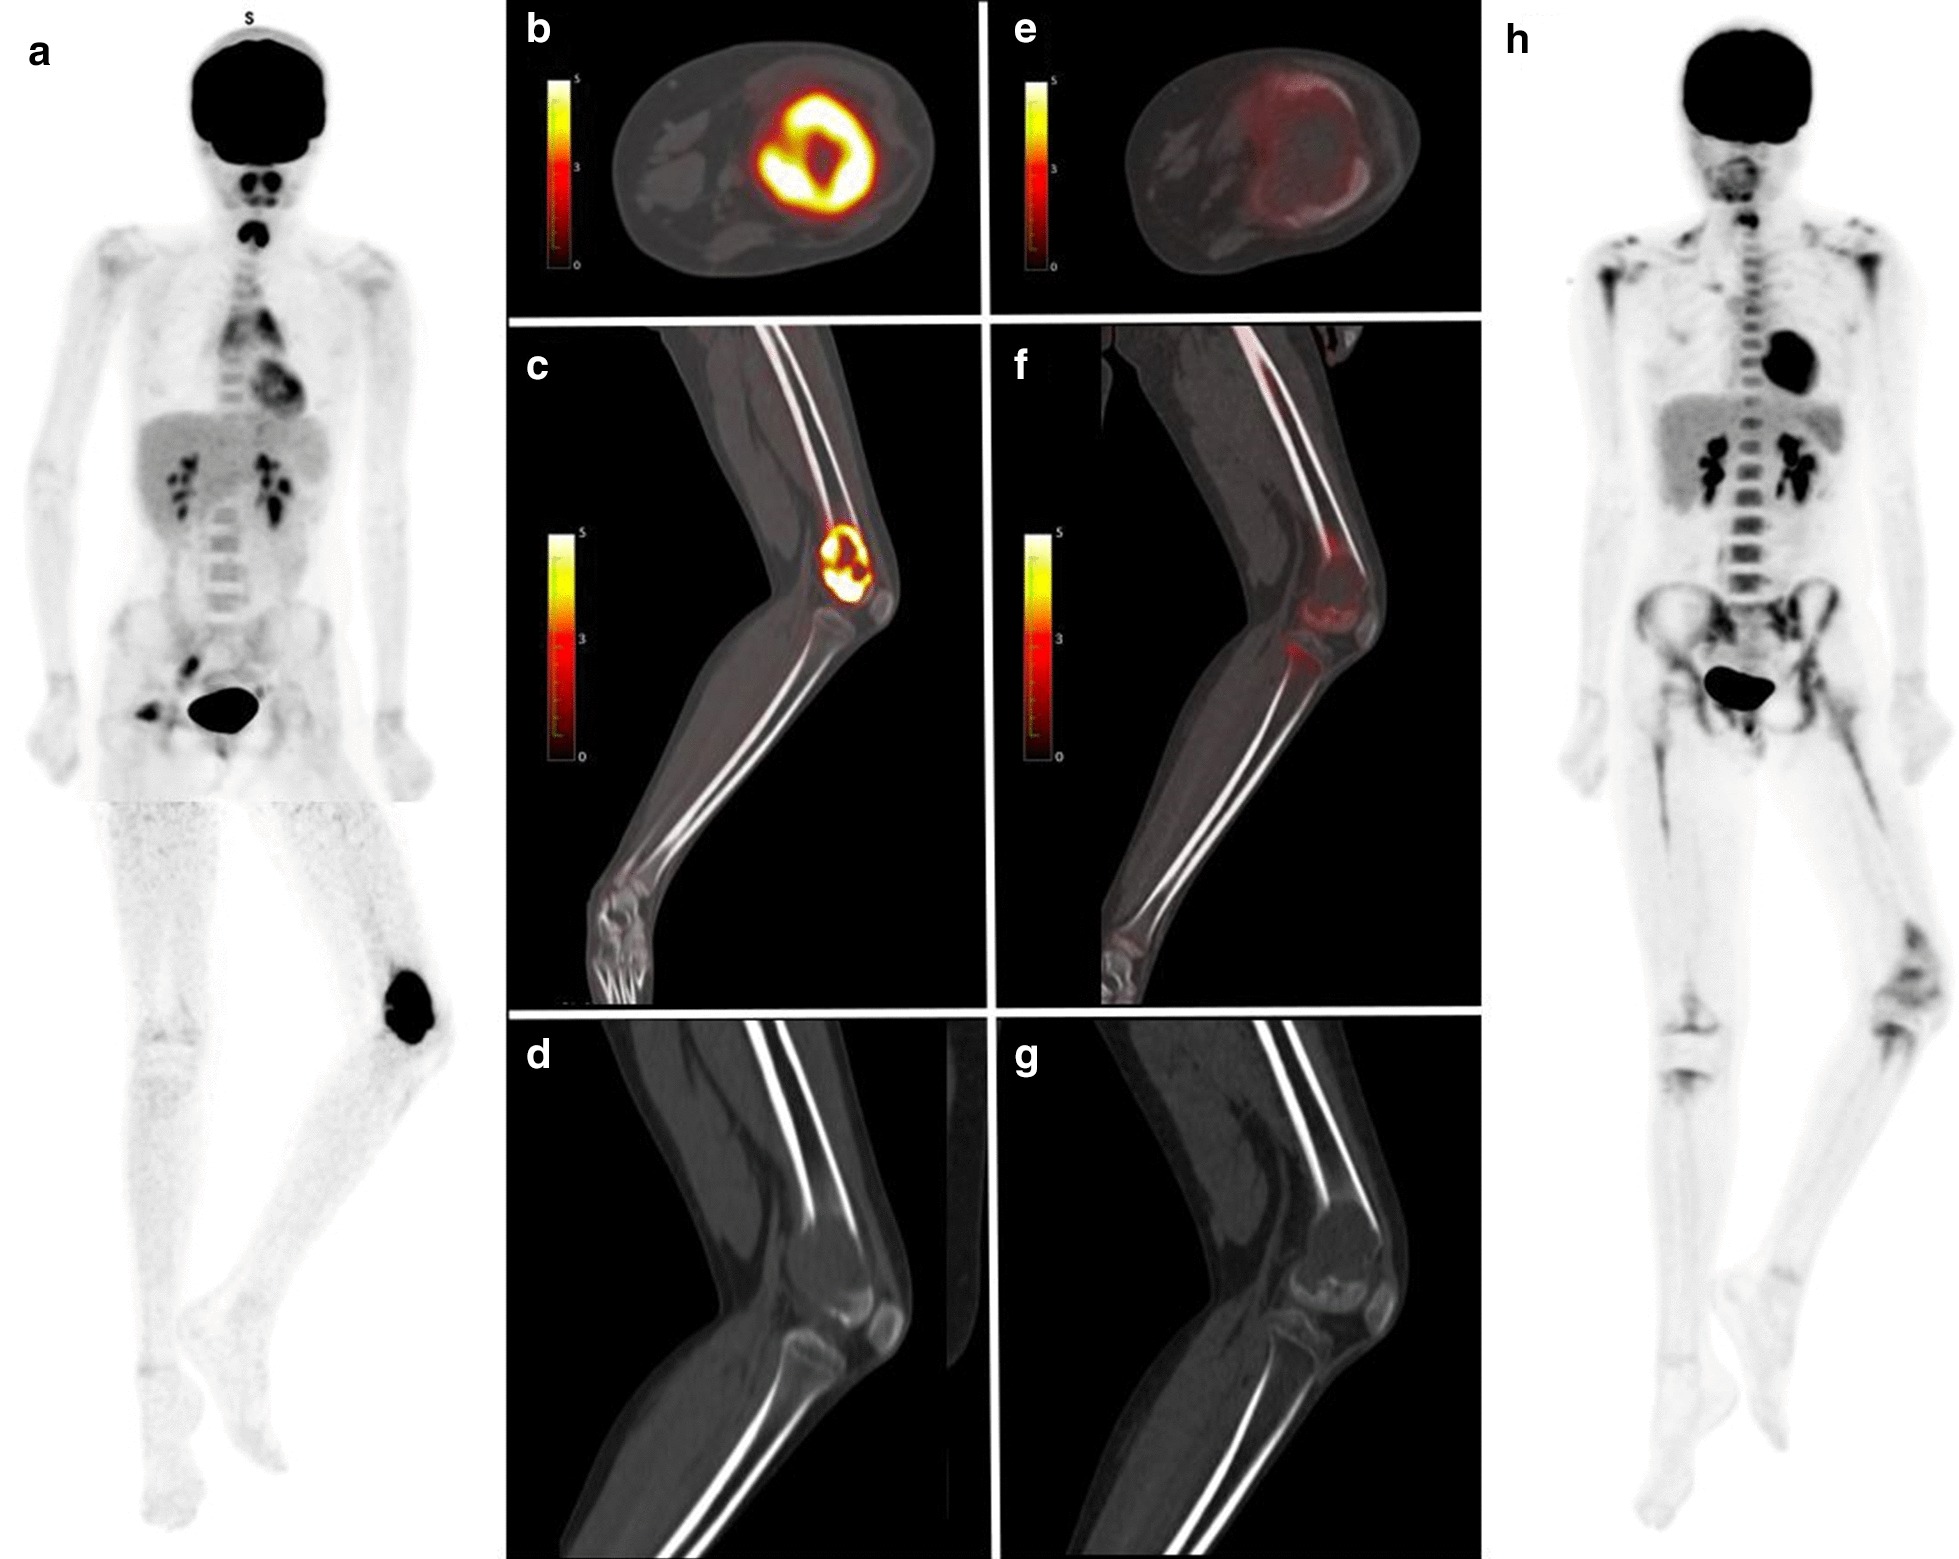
Eleven-year-old girl diagnosed with left distal femur osteosarcoma. PET-1 (a–d) showed stage IIa localized [18F]FDG avid disease (SUVmax 15.2; MTV 113.2 mm^3^; TLG 84.4). Non sarcoma related [18F]FDG uptake was visualized in the thymus (physiologic), the right adnexa (physiologic) and the right piriformis muscle (functional or strain). PET-2 (e–h) showed decreased [18F]FDG uptake (SUVmax 2.2; MTV 83.9 mm^3^; 46.1) after neo-CTX. Diffuse [18F]FDG uptake in bone marrow (h), was related to rebound post-CTX. Patient underwent surgery (necrosis > 99%) and had no evidence of disease (NED) at last follow-up. PET1, a PET 3D MIP, b fused PET/CT axial view, c fused PET/CT sagittal view, d CT sagittal view. PET2, e Fused PET/CT axial view, f fused PET/CT sagittal view, g CT sagittal view, h PET 3D MIP
